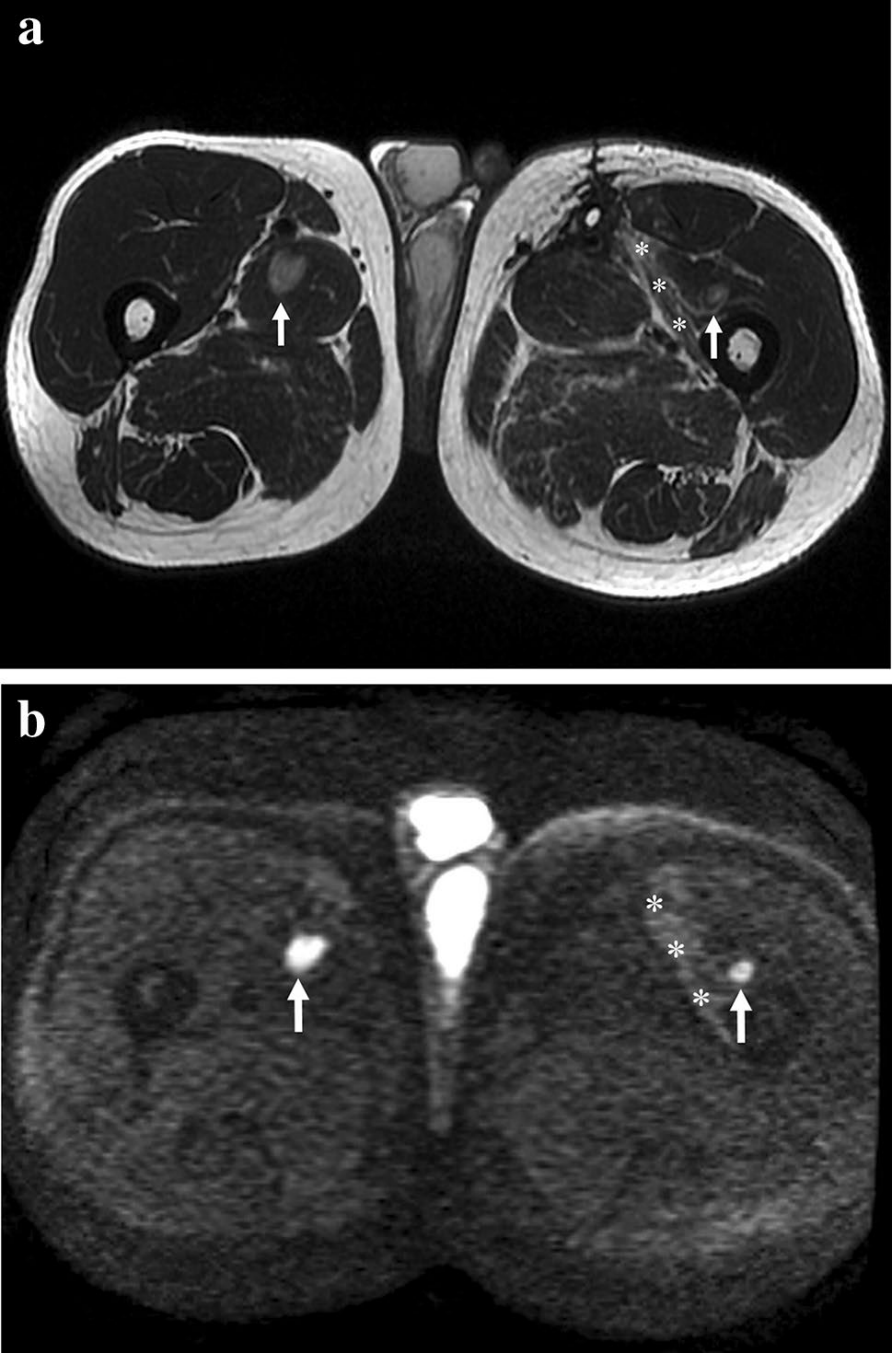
Example of the limits of the quantitative ADC analysis. Sixty-seven-year-old man with recurrent leiomyosarcoma of both proximal thighs. a Axial T2w image shows two hyperintense nodules (*arrows*) in the medial and anterior compartments of the right and left thigh, respectively. b Axial DWI confirms the two nodular lesions (*arrows*) exhibiting bright signal. ADC values of the right lesion (*arrow*) and the scar (*asterisks*) are 1.5 × 10^–9^ m^2^/s and 1.9 × 10^–9^ m^2^/s, respectively
